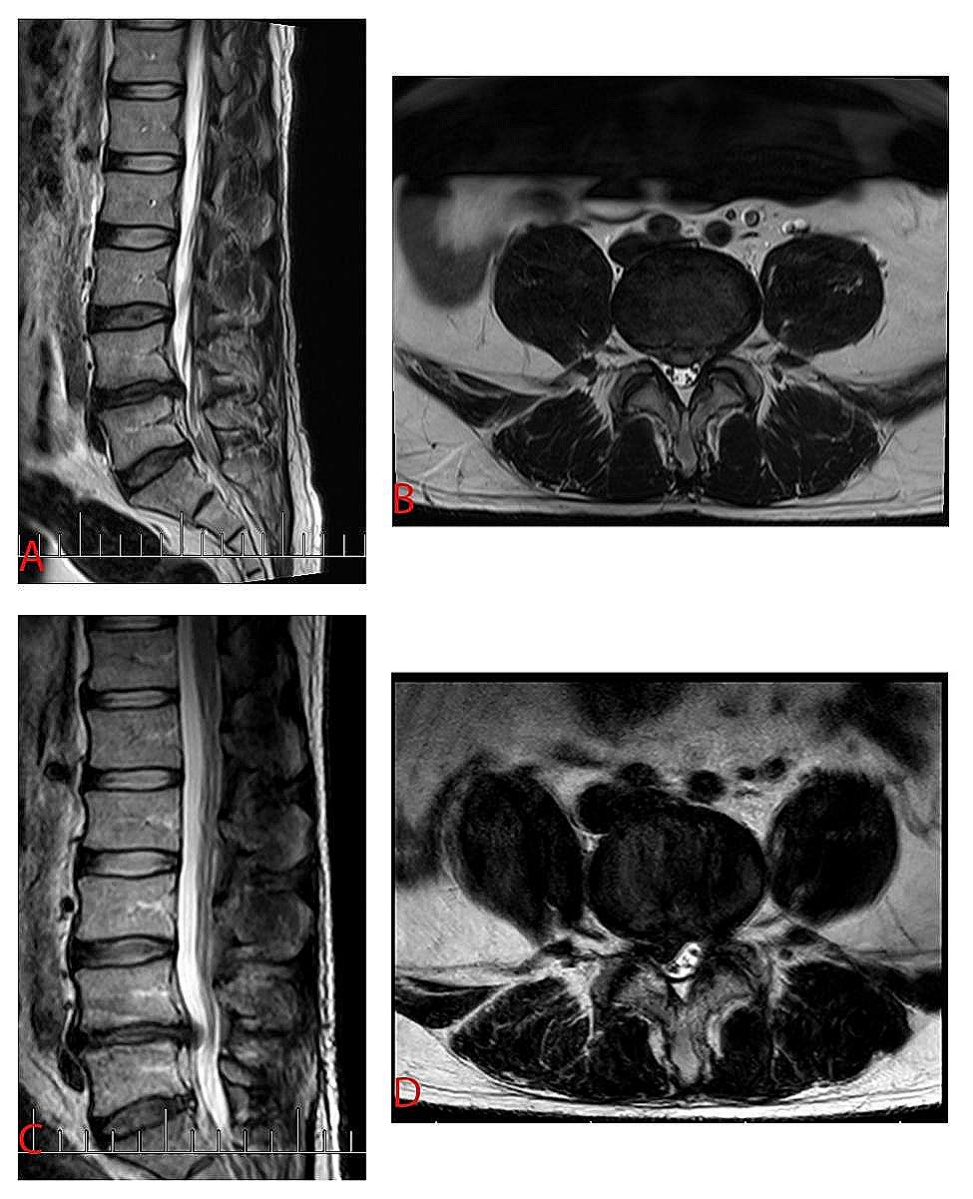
MRI images shows LDH and re-herniation in the patient. *Note*. (A) T2-weighted sagittal lumbar MRI shows LDH at L4-L5 segment. (B) Cross section of L4-L5 segment on T2-weighted MRI shows LDH. (C) T2-weighted sagittal lumbar MRI shows re-herniation at L4-L5 segment after discectomy by spinal endoscopy through the transforaminal approach. (D) Cross section of L4-L5 segment on T2-weighted MRI shows re-herniation
